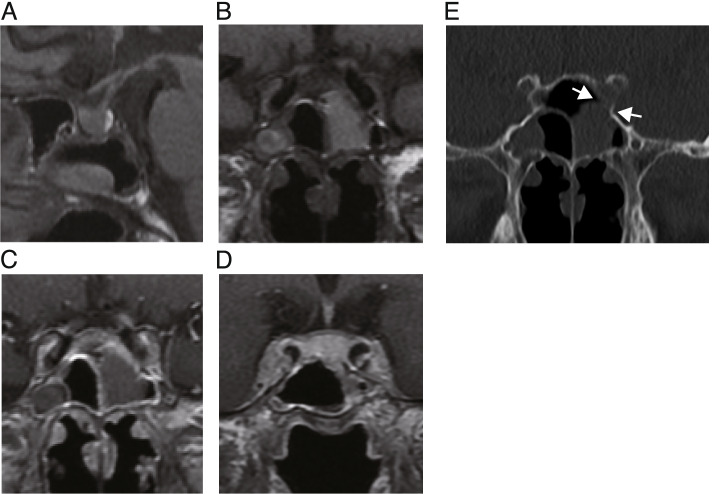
MRI A-D and CT E images at the first admission. C, D MRI performed after injection of gadolinium. E Space between arrows indicates the erosion of the sphenoidal bone
